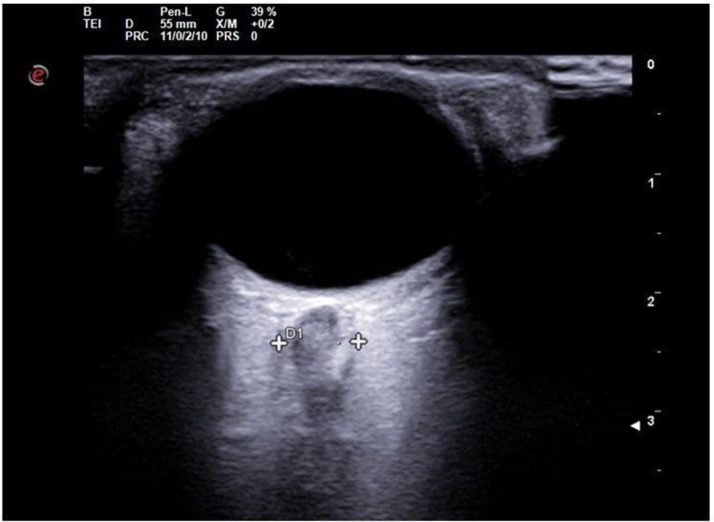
Measurement of ONSD using grayscale ultrasonography. ONSD = optic nerve sheath diameter.
